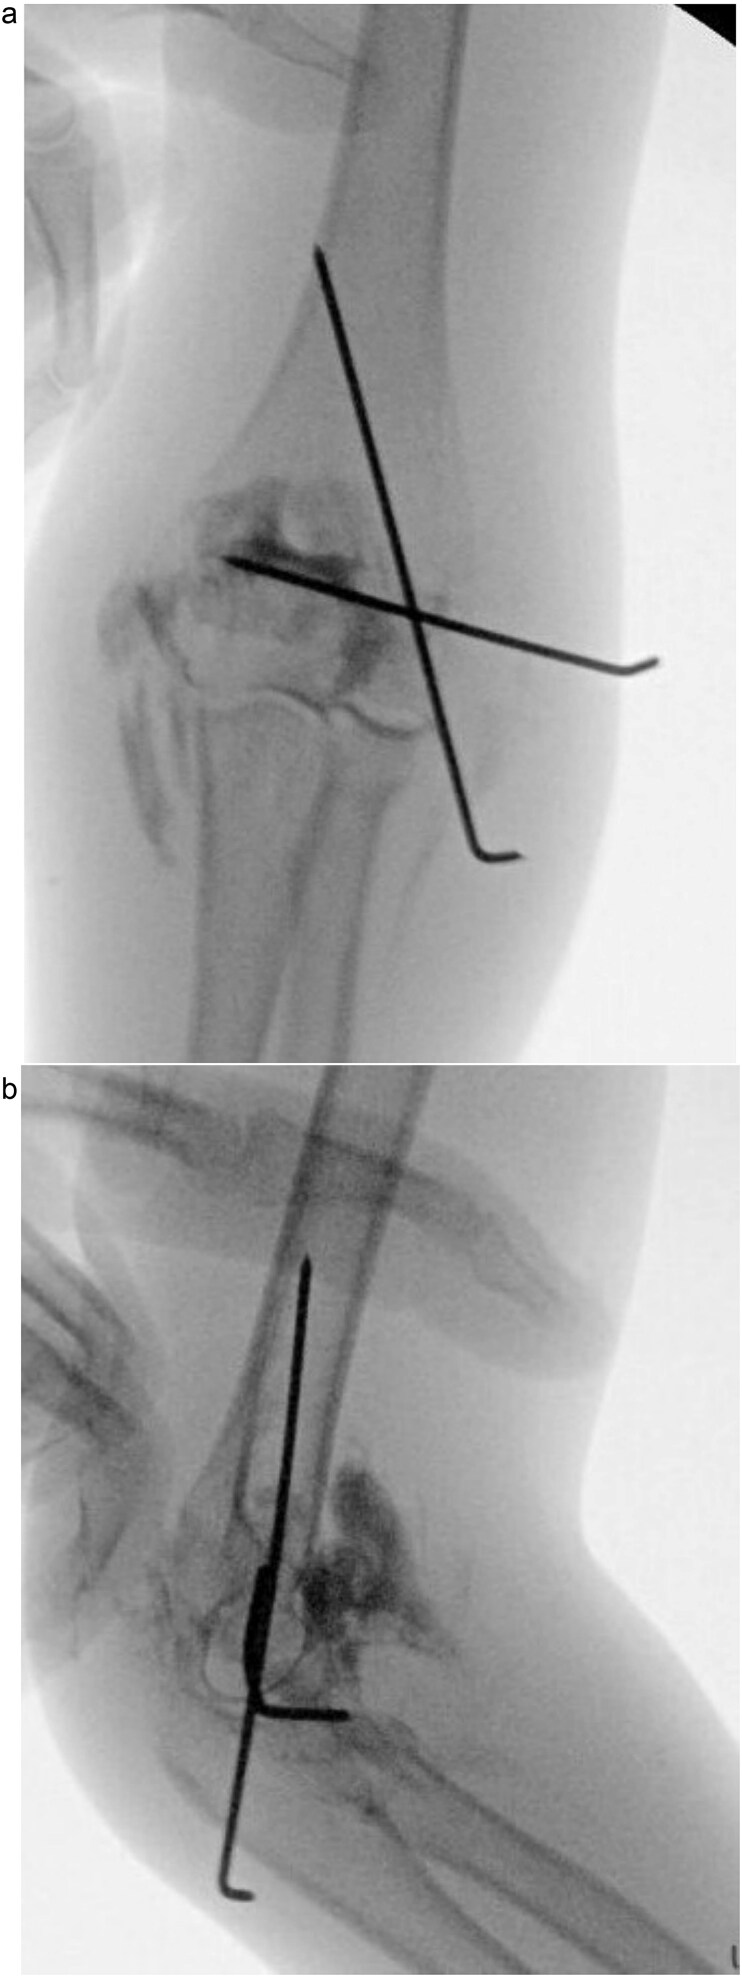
(a) Postoperative anteroposterior radiograph demonstrating stable fixation of the lateral condyle with two smooth Kirschner wires. (b) Lateral view showing proper wire placement and a well-aligned elbow maintained in a posterior slab splint.
